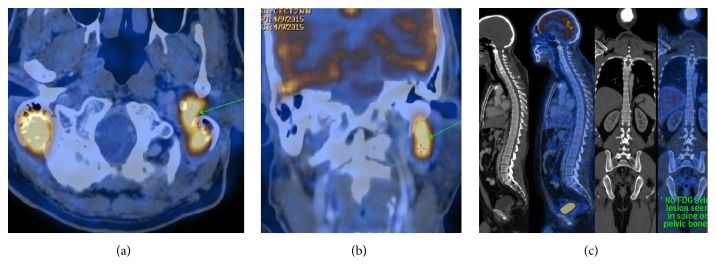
Whole body PET-CT scan revealed FDG-avid lesions (SUV max. 3.6) in both mastoids and along left styloid process (a, b). No other FDG-avid lesions were detected in the rest of the body (c).
